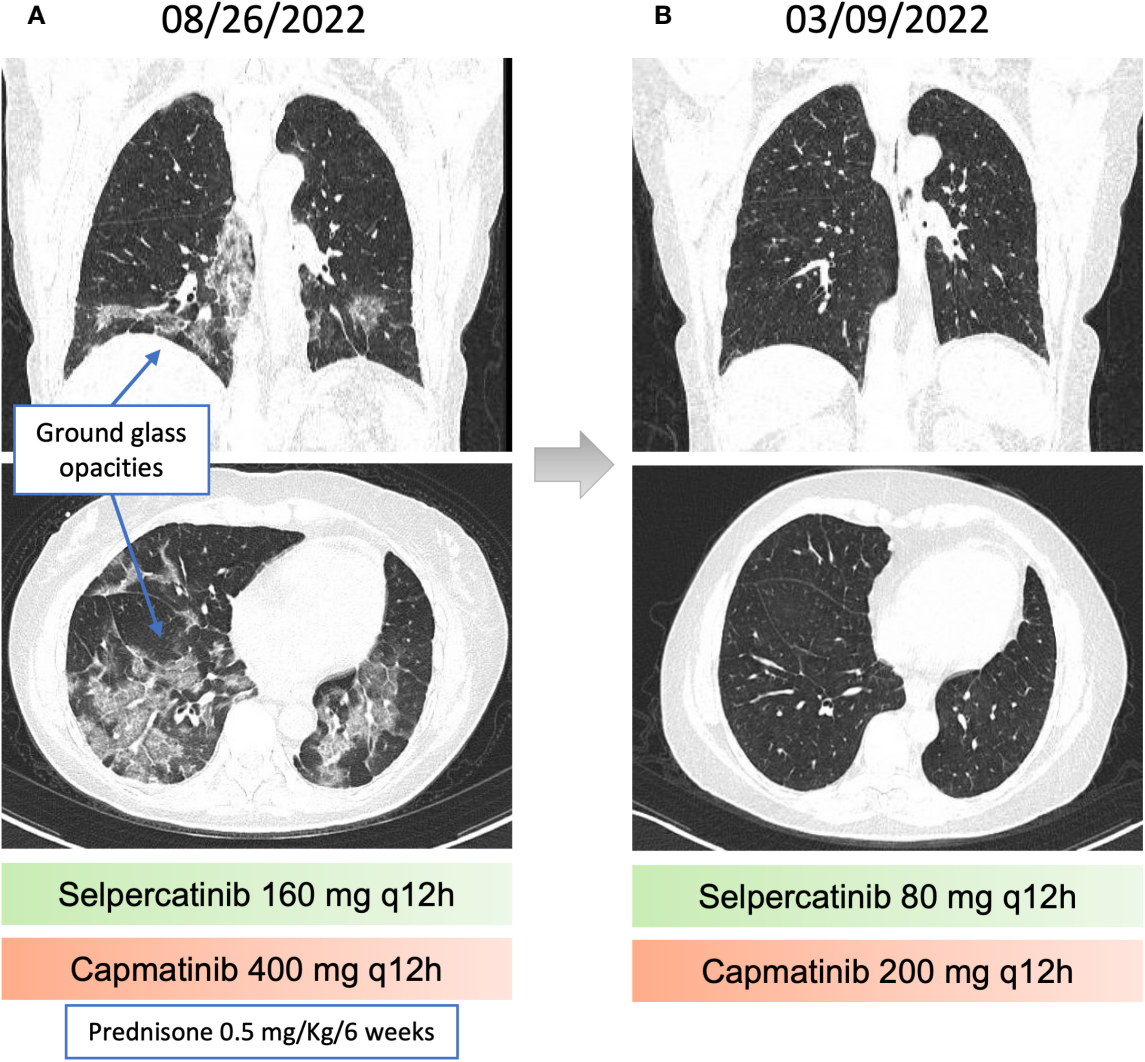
Pneumonitis related to capmatinib and recovery after dose reduction. (A) chest computed tomography (CT) revealing Ground-glass opacities, associated with perilobular consolidation components, distributed in peribronchovascular and peripheral regions, suggestive of organizing pneumonia. (B) Resolution of the findings described in the previous chest CT.
